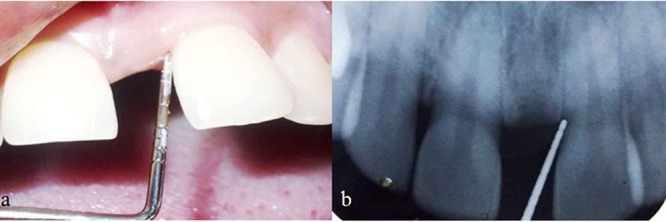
a: Clinical healing of treated defect 2 years postoperative showing 0.5mm probing depth only.  b: 2 years postoperative radiographic view of tooth #21 region
